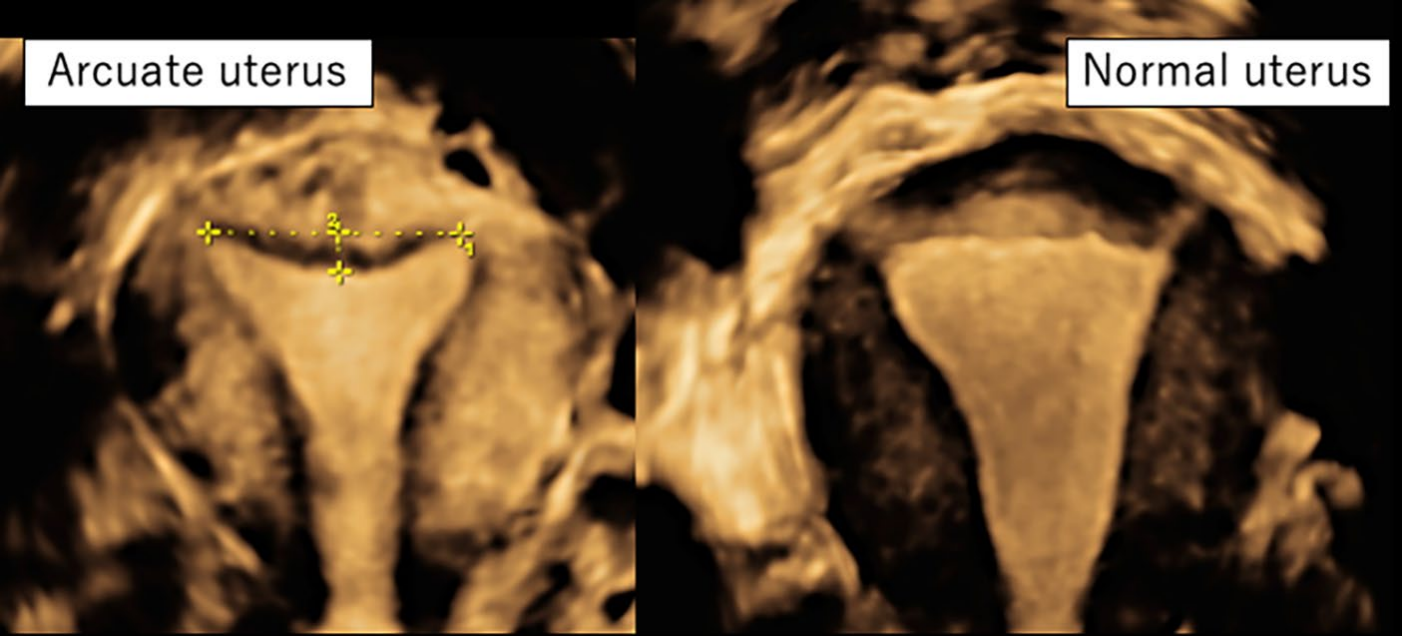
Classification of Arcuate uterus and Normal uterus based on 3D vaginal ultrasonography. Three‐dimensional ultrasound of an arcuate uterus showed a uterine cavity that was concave and a broad focal thickening of the myometrium < 1 cm in length from the inter‐osial line. Normal uterus showed that the uterine cavity was straight or convex.
